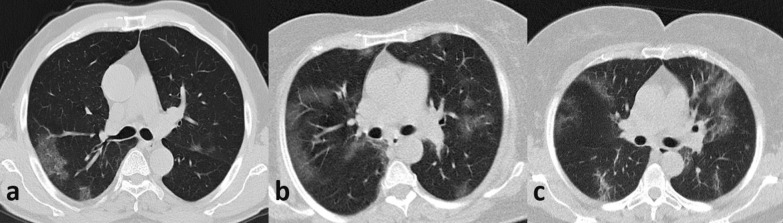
Example cases of three patients with typical findings of COVID-19 in FDCT (a) and LDCT (b, c). Adequate image quality is obtained both in full-dose and ultra-low dose CT chest protocols. a FDCT—normal BMI—DLP: 192 mGy*cm. b LDCT—normal BMI—DLP: 20 mGy*cm. c LDCT—Elevated BMI—DLP: 26 mGy*cm
